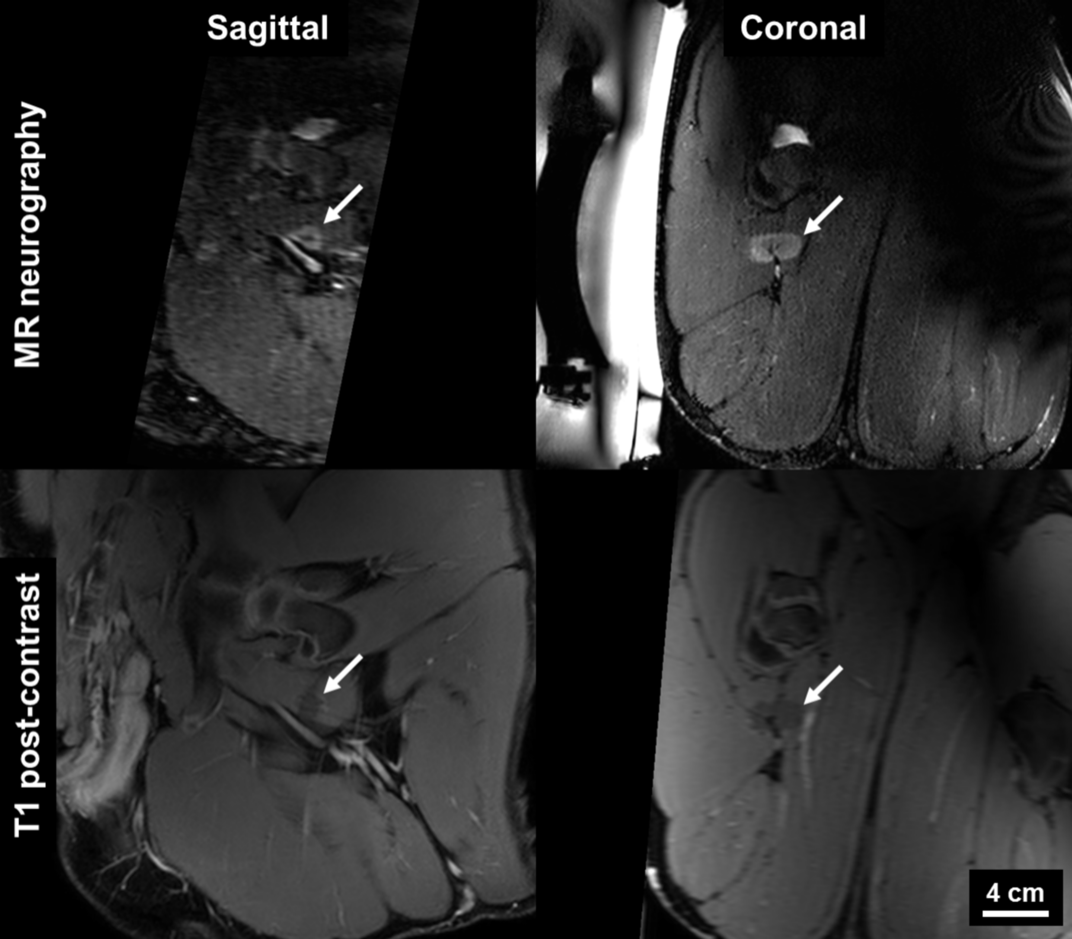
MRI evaluation of thermal lesions in peripheral nerve. Hyperintense region on 3D diffusion-prepared MR neurography (top) corresponds with non-enhancing region on T1 post-contrast image.
